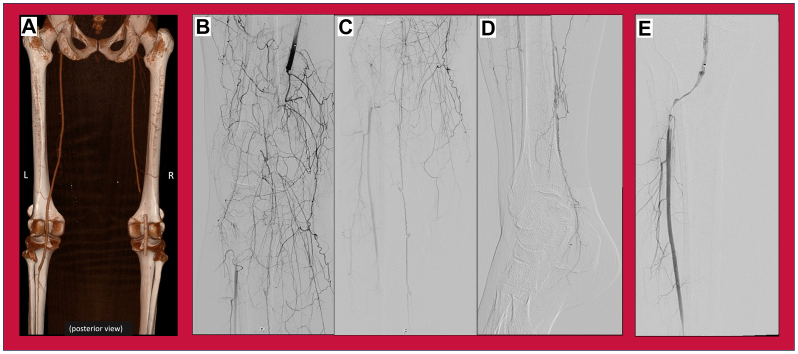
Initial computed tomography angiography (CTA) and angiogram, pre- and post-thrombolysis, during the index encounter for right acute limb ischemia. (A) Reconstructed three-dimensional CTA runoff posterior view showing occluded right popliteal artery proximal to stent; (B-D) Pre-intervention angiogram of the right leg; (E) post-thrombolysis angiogram of the right leg.
